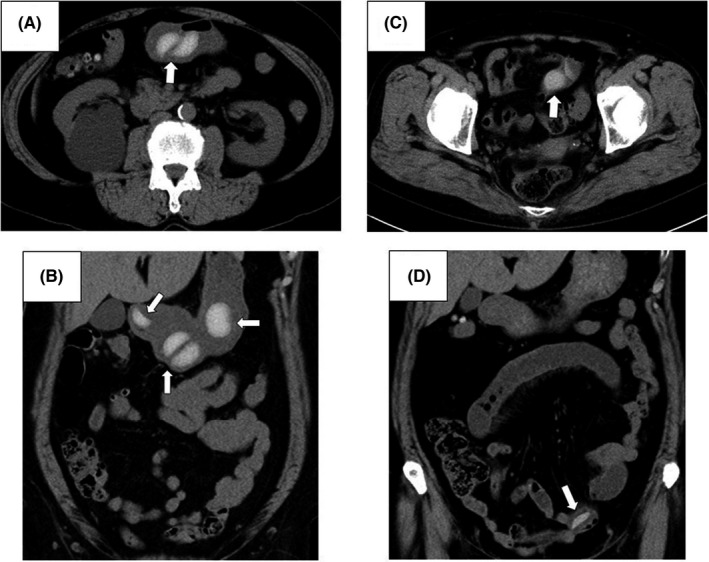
(A) Axial section of abdominal plain CT scan shows (arrow) high‐density intraluminal ingested food item (rice cakes) in stomach. (B) Coronal reformation of abdominal plain CT scan shows (arrow) high‐density bodies (rice cakes) in stomach. (C) Axial section of abdominal plain CT scan shows (arrow) high‐density body (rice cake) in ileum. (D) Coronal reformation of abdominal plain CT scan shows (arrow) obstructing high‐density body (rice cake) in ileum with dilation of ileum proximal to obstruction
